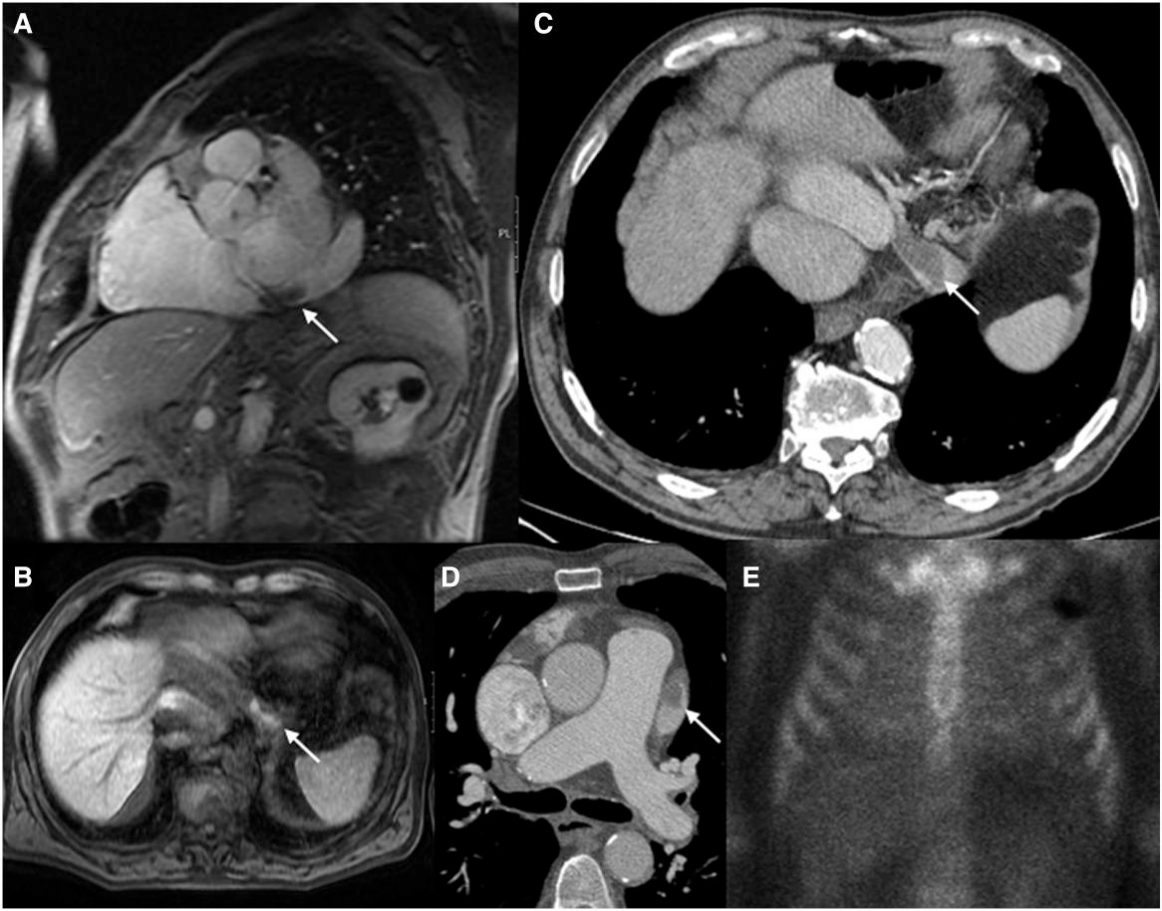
Cardiac magnetic resonance, computed tomography, and 99Tc-DPD scintigraphy. Dilatation of coronary sinus with thrombosis inside (arrow) in short-axis view (*A*); hyper-intensive view (arrow) in pre-contrast sequences in the coronary sinus consistent with early thrombus (*B*). Images of computed tomography showing coronary sinus thrombosis (arrow) in portal phase (*C*) and thrombosis (arrow) in left atrial appendage (*D*). Image of cardiac technetium-99m-labelled 3,3-diphosphono-1,2-propanodicarboxylic acid (99Tc-DPD) scintigraphy shows slight uptake (grade 1) of labelled disphosphonates in the myocardium with diffuse distribution (*E*).
